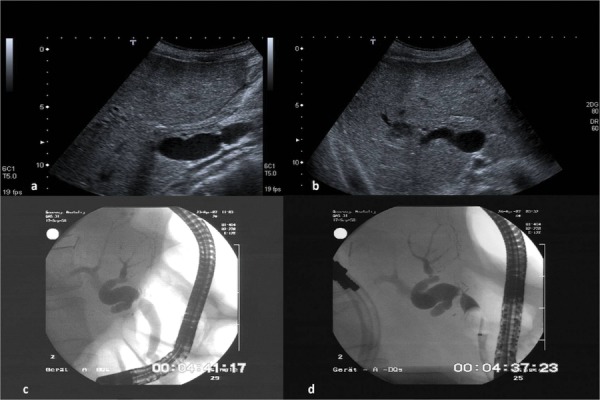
The Images Show a Stenosis at The Level of The Anastomosis With Intrahepatic Dilatation of The Bile Ducts Figure 1a demonstrates a liver recipient with a biliodigestive anastomosis in a longitudinal position of the ultrasound probe in the media clavicular line. Dilatated gut loop with fluid in liver hilum. Figure 1b demonstrates the right lobe of the same patient in a sub costal view. Dilatated right common bile duct with a peripheral abscess (arrow) due to the stenosis of the bile duct at the level of the bilio digestive anastomosis. Figure 1c and 1d demonstrate the corresponding ERCP images without and with blocked balloon catheter
